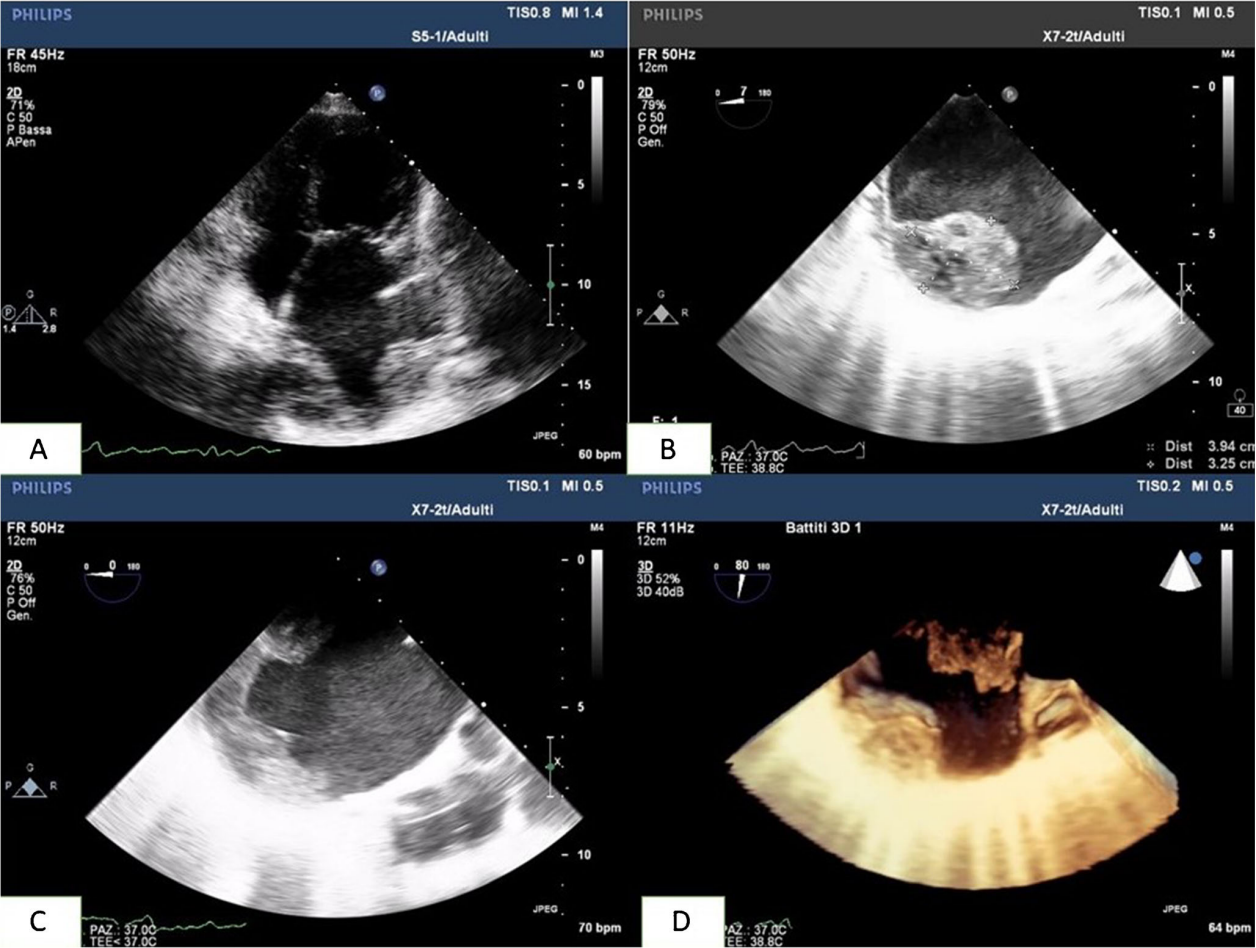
Transthoracic (A) and transesophageal (B, C, D) echocardiography showing left atrial thrombotic formation
